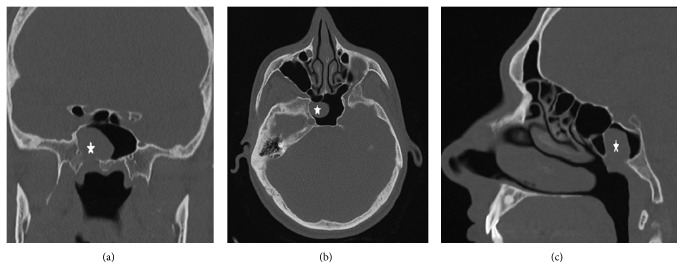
Computed tomography image of angiofibroma localized in the sphenoid sinus ((a) coronal, (b) axial, and (c) sagittal).
